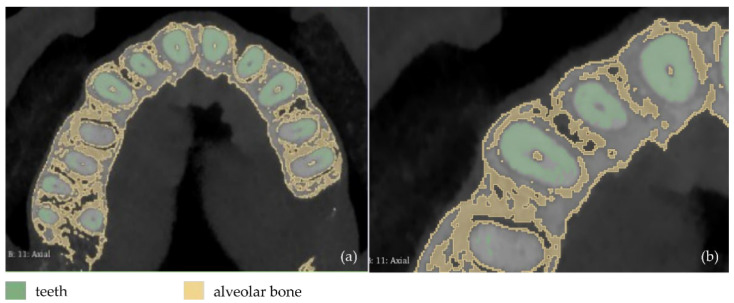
Automatic thresholding results for the segments defined for the teeth and the alveolar bone (Experiment 3); (a): a CBCT slice in the axial plane; (b): zoom-in view of (a).
